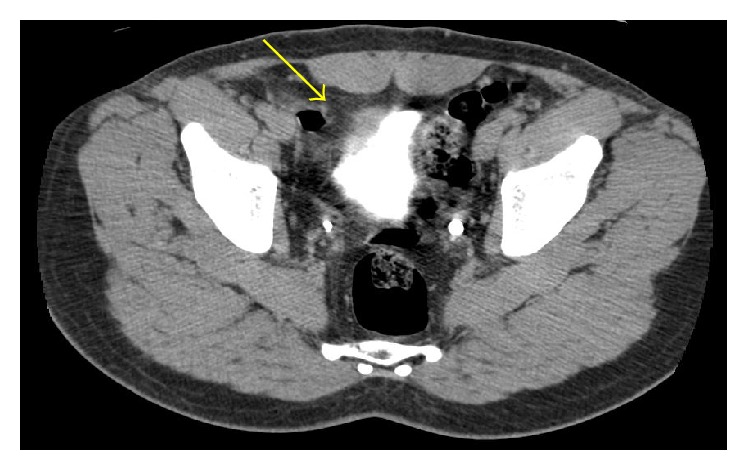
CT-scan of the second case showing a bladder wall thickening, with contrast enhancement.
